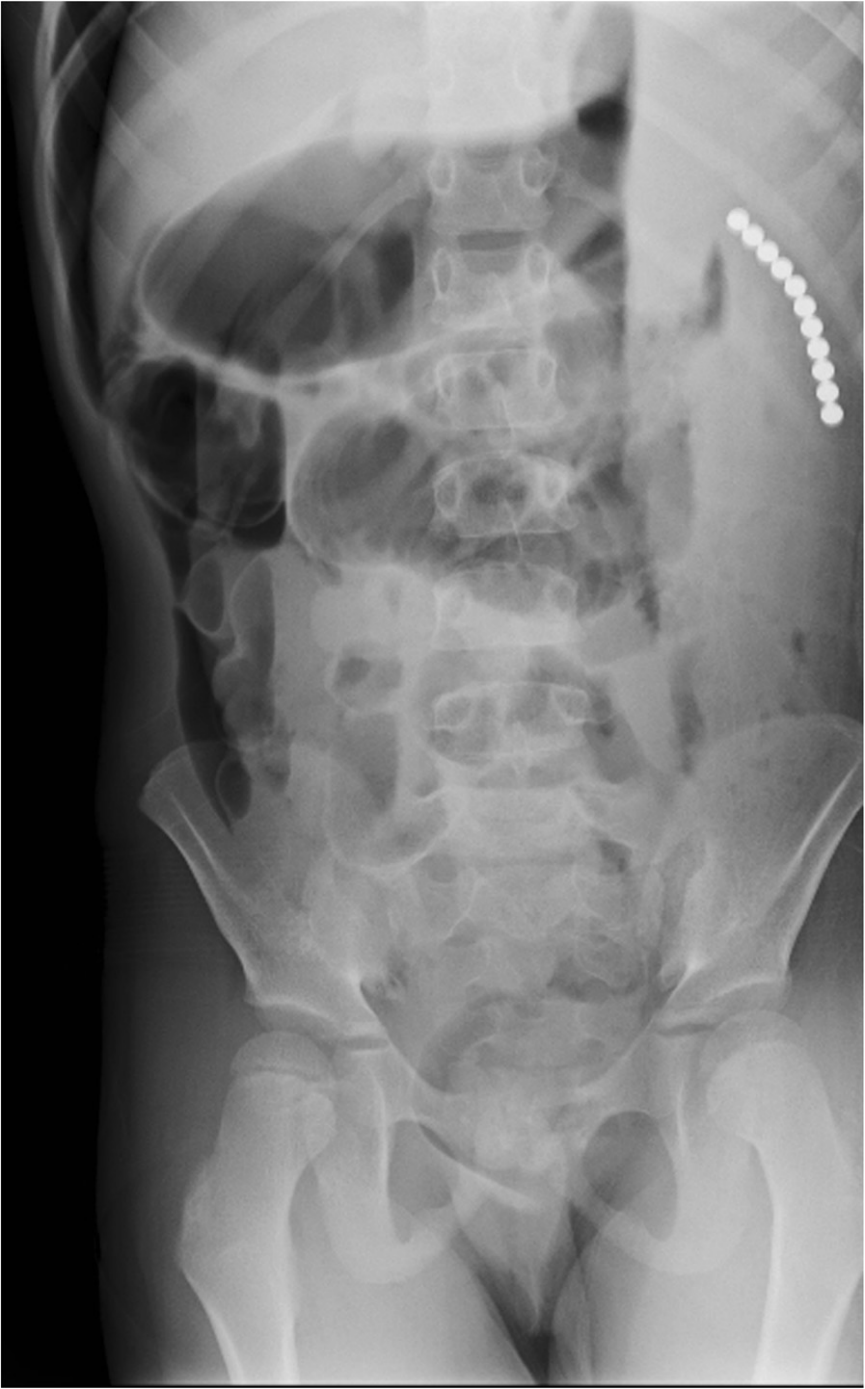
Left lateral decubitus radiograph obtained in a 5 year-old boy with the new complaint of abdominal pain the morning after an MRI of neck was performed for complaint of neck pain. Radiograph demonstrates 11 round metallic objects 4 mm in diameter and pneumoperitoneum.
